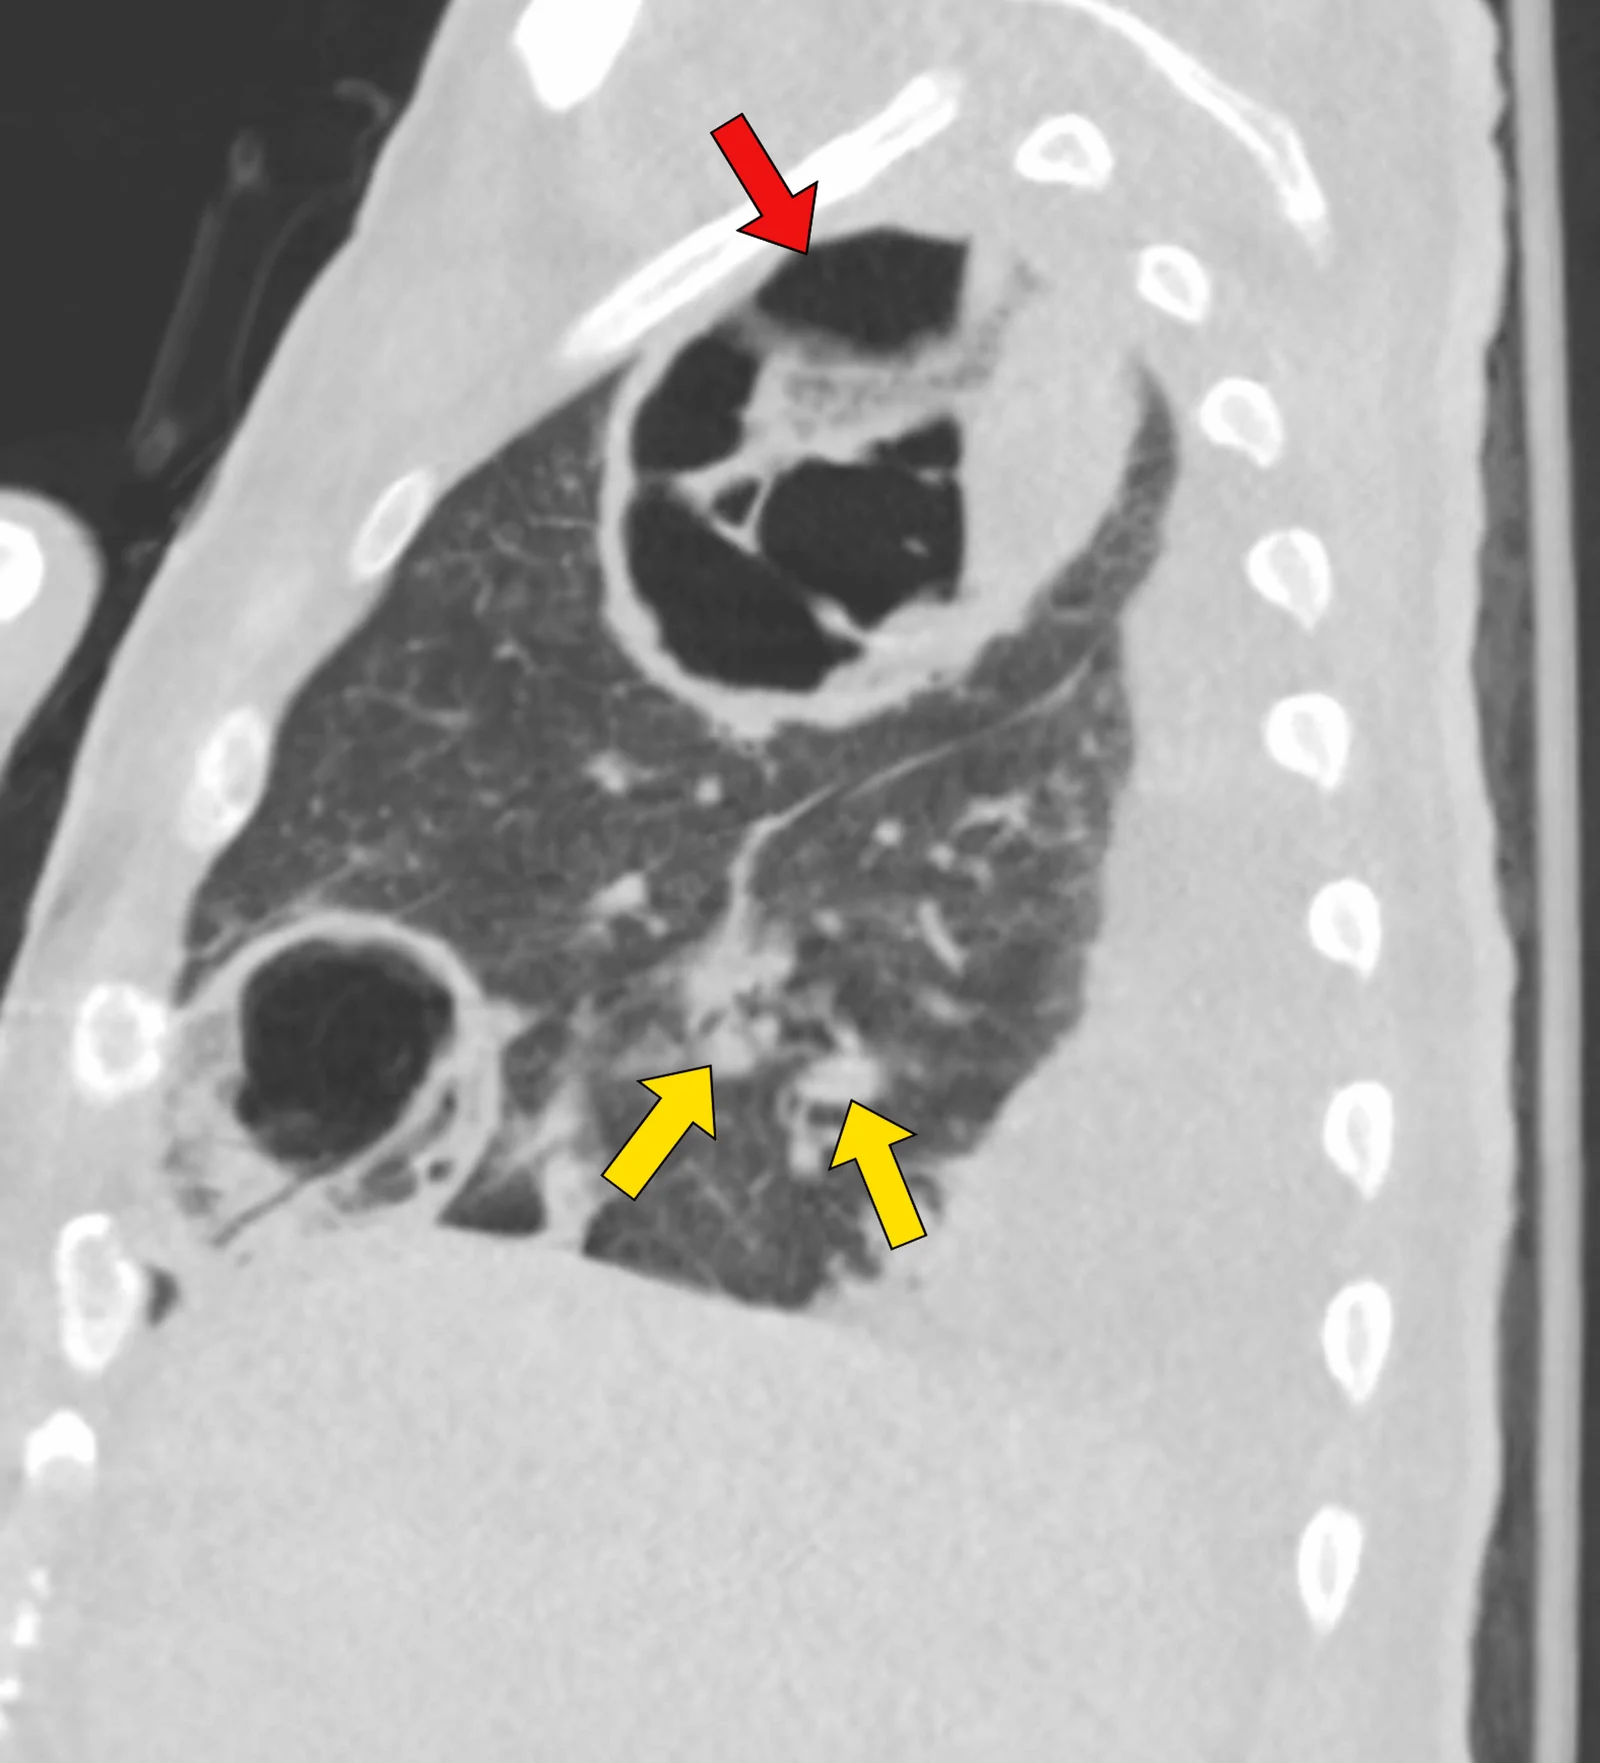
Sagittal CT image of the chest demonstrates the cavitary lesion (red arrow) and pulmonary nodules (yellow arrows). The extensive cavitary disease raised concern for an invasive infectious process in addition to the initially identified bacterial pneumonia.
